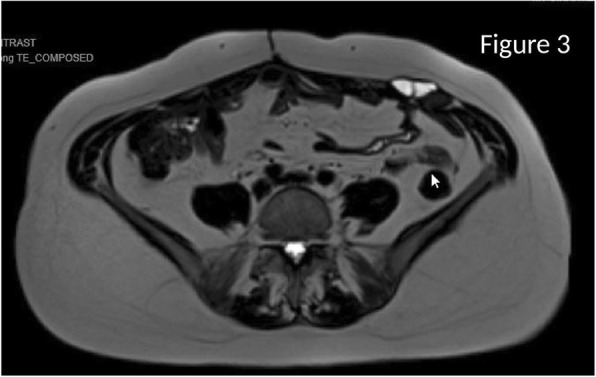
Surveillance MRI of the abdomen/pelvis with no evidence of metastatic disease
